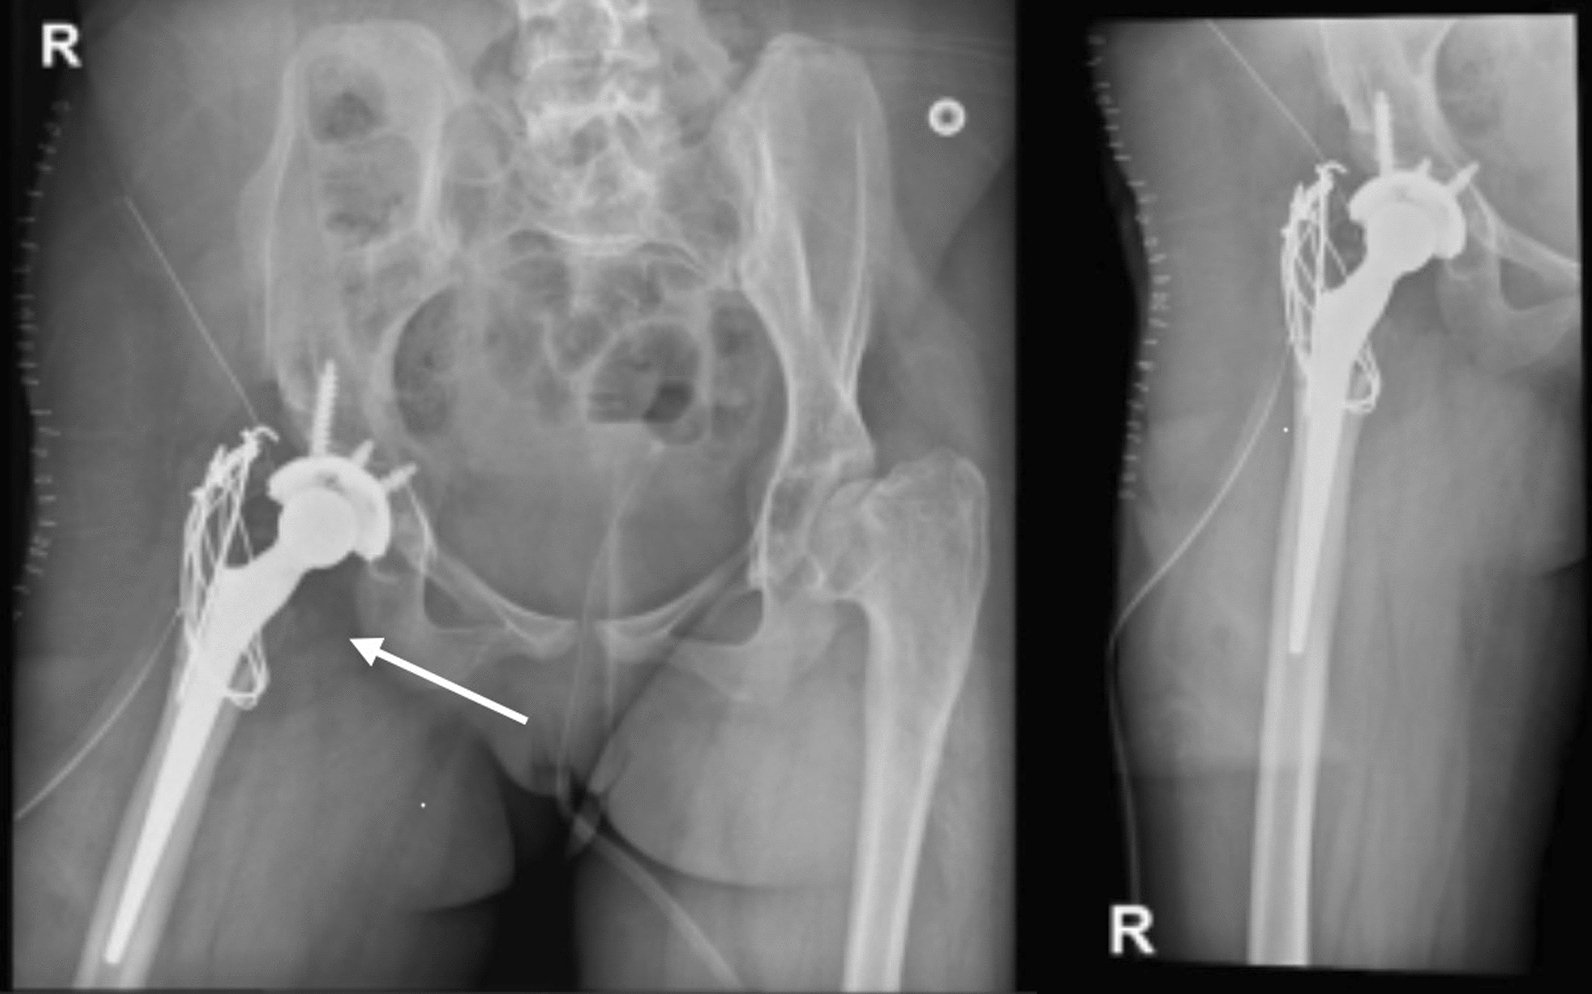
X-ray of right hip showed the implant well positioned and with good alignment and there is no obvious osteolysis or dislocation or any pressure was seen on the right hip
